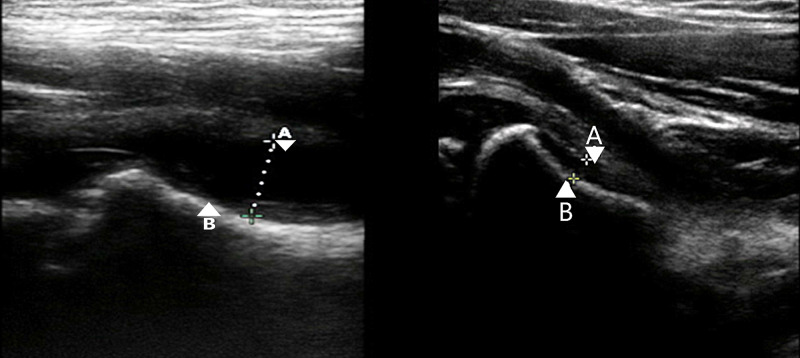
Ultrasound demonstrating a hip joint effusion in a paediatric patient is shown on the left. For comparison normal physiological joint fluid volume is shown on the right. Ultrasound images from Pade et al., 2020 [16] A – Capsule of hip joint B – Bony cortex of femur Dotted line - Depth of hip effusion Original images authorised by respective owners
